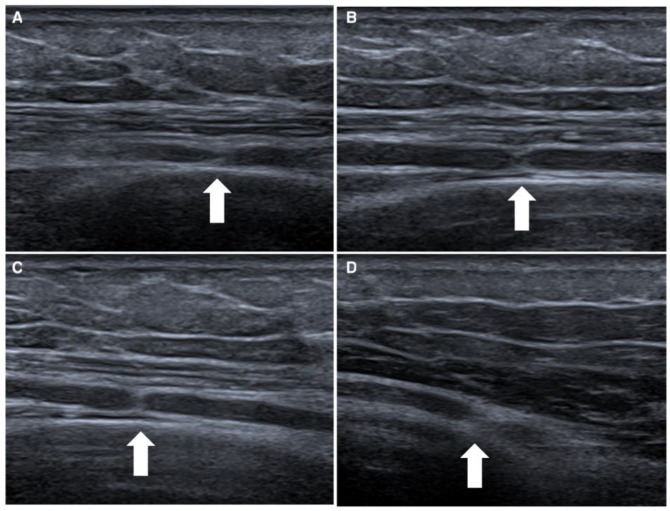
Ultrasonographic appearance of first case, a rare case of quadruple torsion of the radial nerve. In this figure is represented the radial nerve with complete constriction of four nerves, which are located 10 cm (white arrow in box (A)), 8 cm (white arrow in box (B)) and 4 cm above the elbow crease (white arrow in box (C)), and at the elbow crease (white arrow in box (D)).
